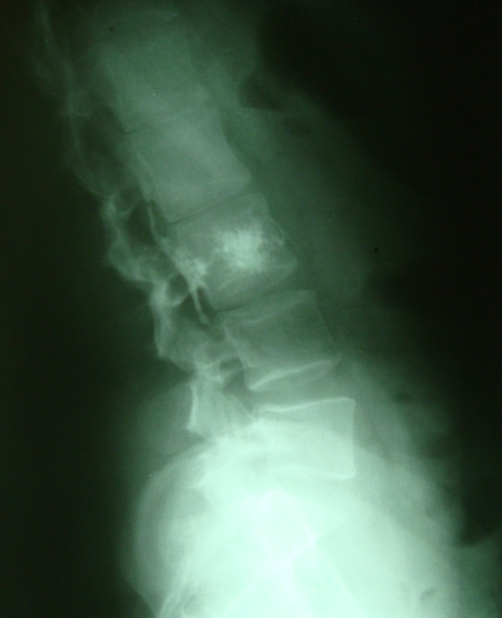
Plain radiograph after vertebroplasty. Posterior cement leakage is obvious.
